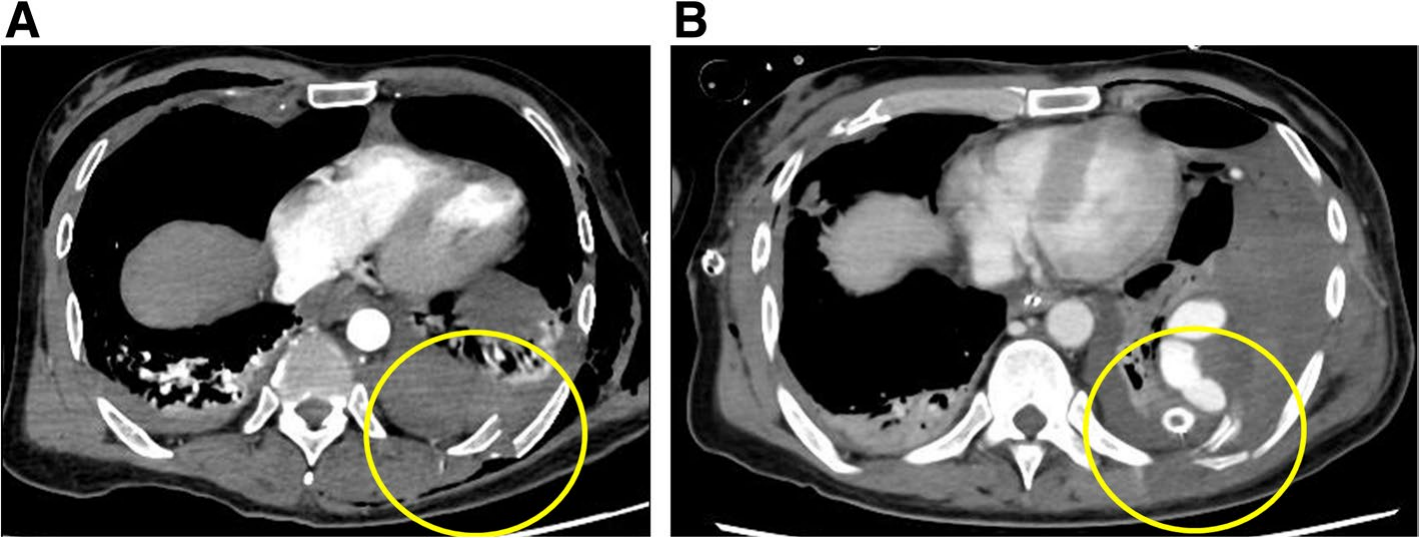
A Chest computed tomography before the initial surgery (yellow circle: 9th rib). B Chest computed tomography before the second surgery (yellow circle: 9th rib)
